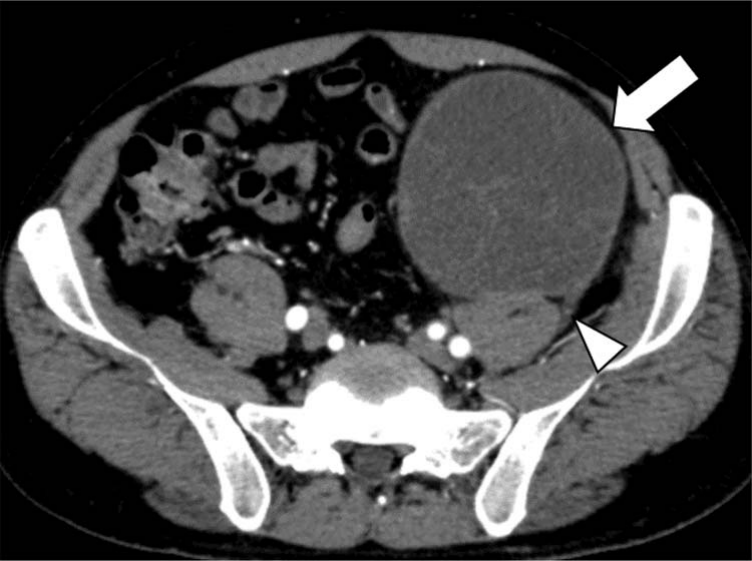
Contrast-enhanced CT confirmed a 10-cm hypodense solid mass (arrow) in the retroperitoneum of the lower left abdomen. It showed that the tumor was contiguous with the third lumbar nerve (arrowhead), suggesting that it was a schwannoma.
